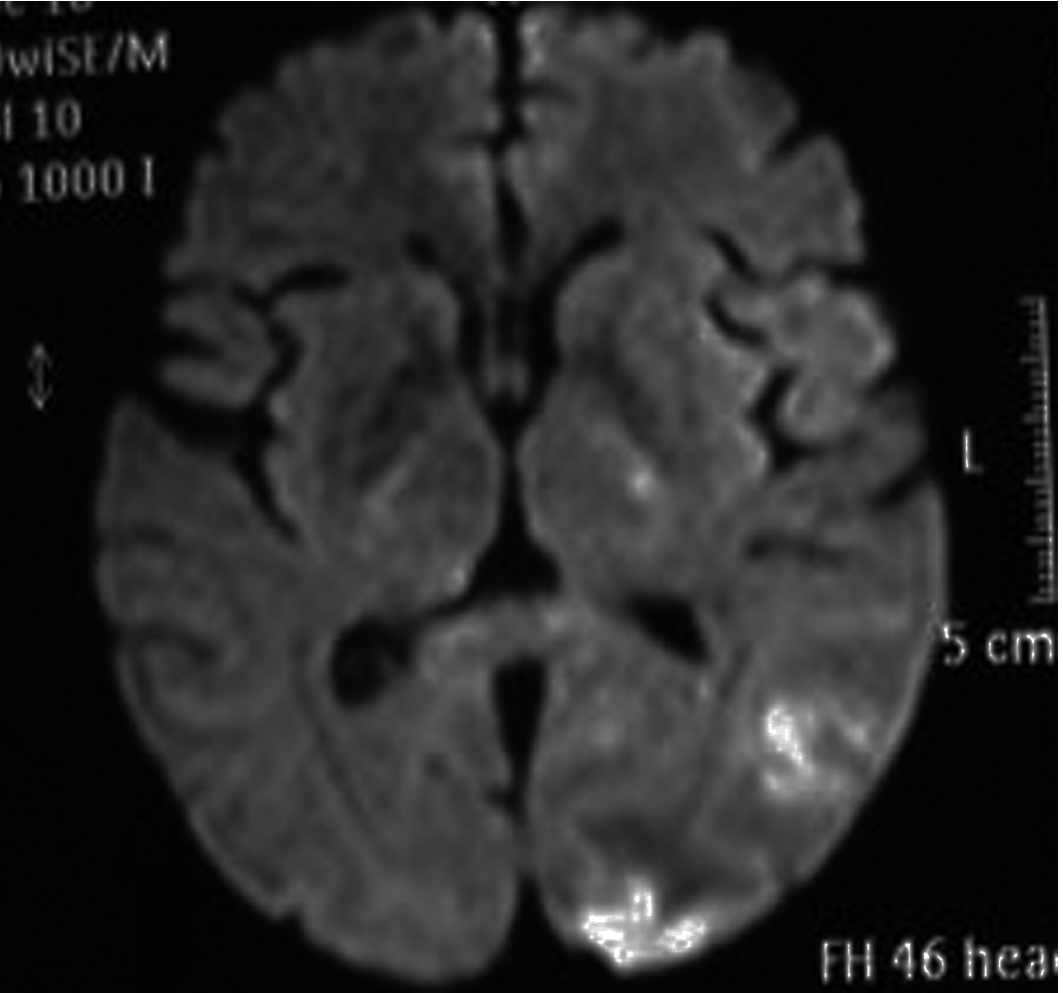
Brain MRI DWI: left occipital cortical restriction in DWI.
